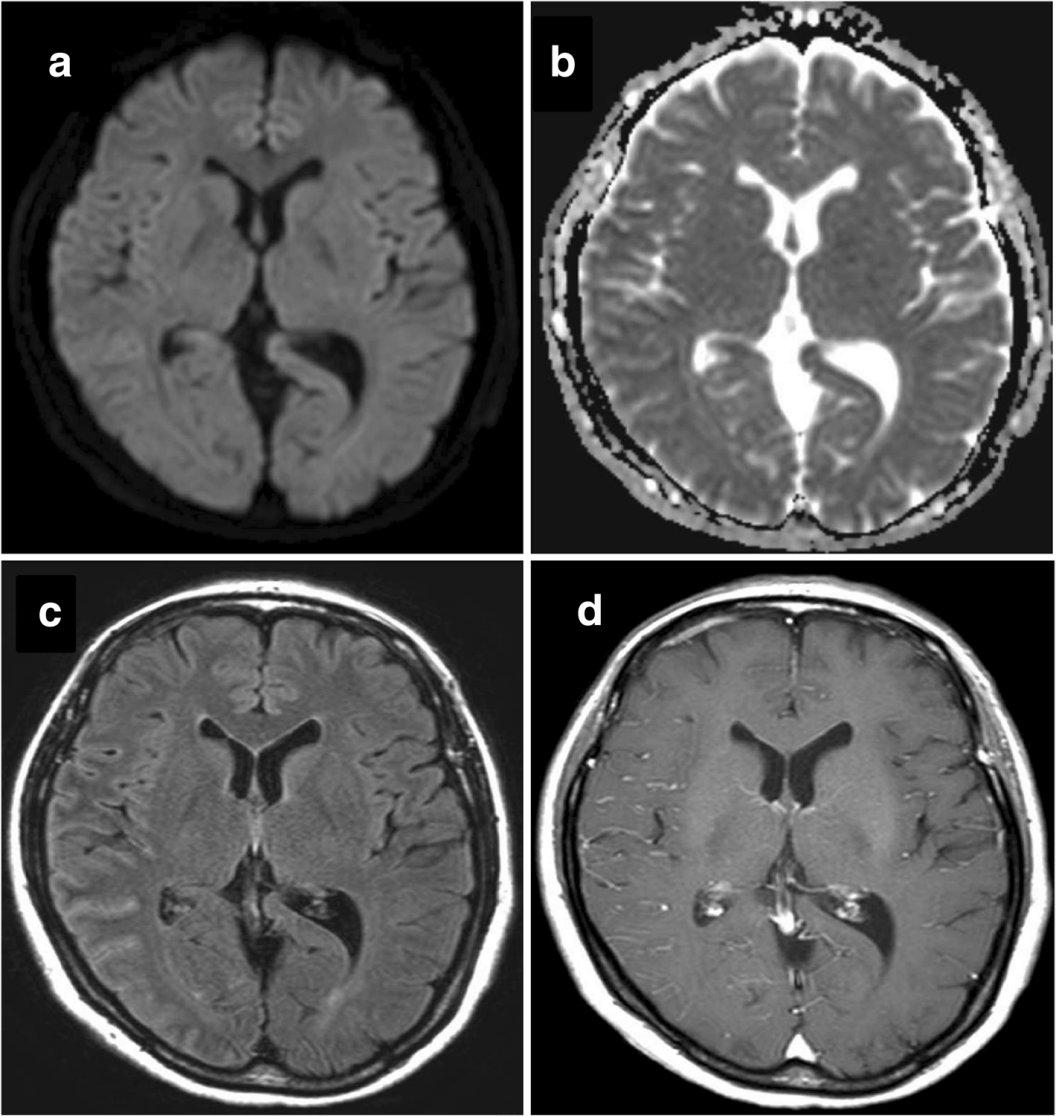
MRI images at day 3. The abnormal findings at day 1 have disappeared on DWI (a) and the ADC image (b), but the high-intensity area remains on the FLAIR image (c) at day 3. There is no definite enhancement of the lesion on gadolinium-enhanced T1-weighted imaging (d). MRI = magnetic resonance imaging, DWI = diffusion-weighted imaging, ADC = apparent diffusion coefficient, FLAIR = fluid attenuated inversion recovery
